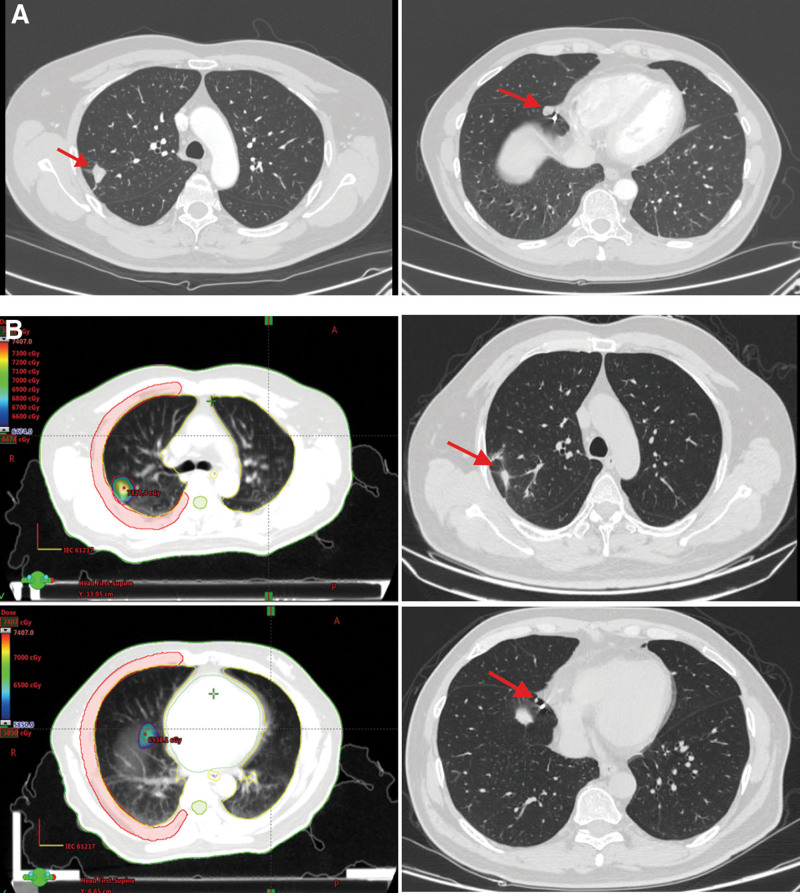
(A) CT of 2 lung metastasis. (B) Radiotherapy plan and CT after radiotherapy of 2 lung metastasis. CT = computed tomography.
